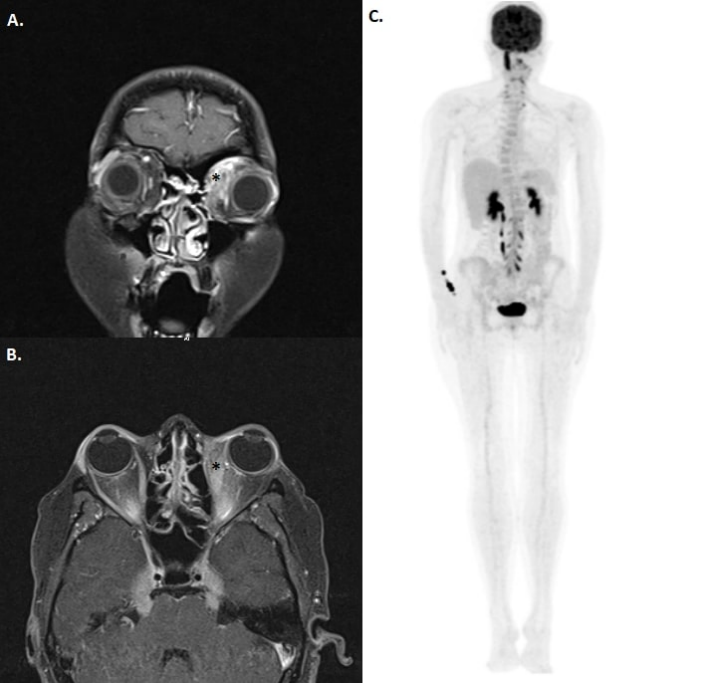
In coronal and axial T1 fat suppression MRI sections, increased contrast retention in left medial rectus and medial orbital soft tissue were observed (right). Increased FDG intake in right wrist, dorsal, lumbar and sacral vertebras was observed at PET-CT (left).
